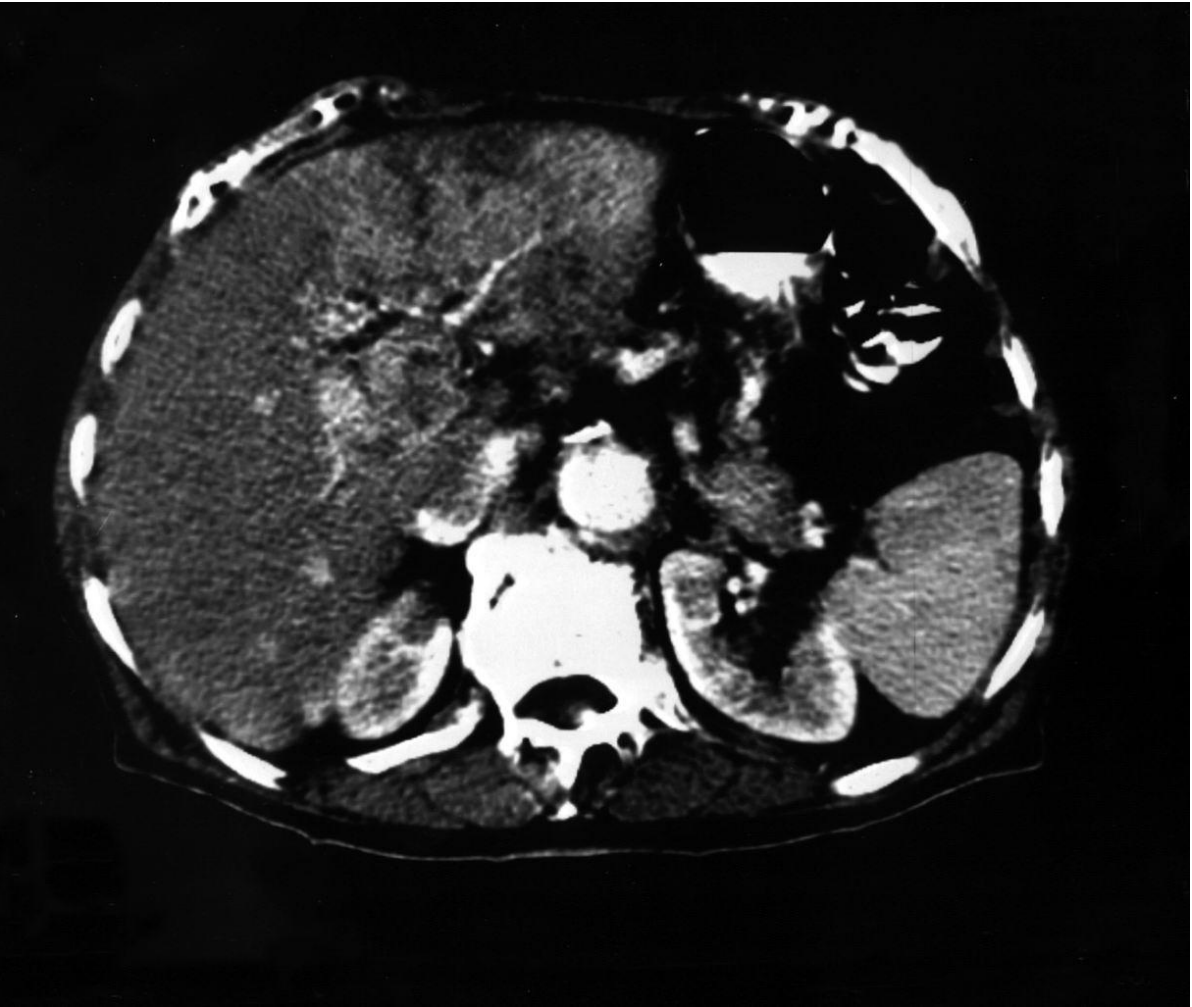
Hyperdense lesions of the liver in the arterial phase of the CT scan.
